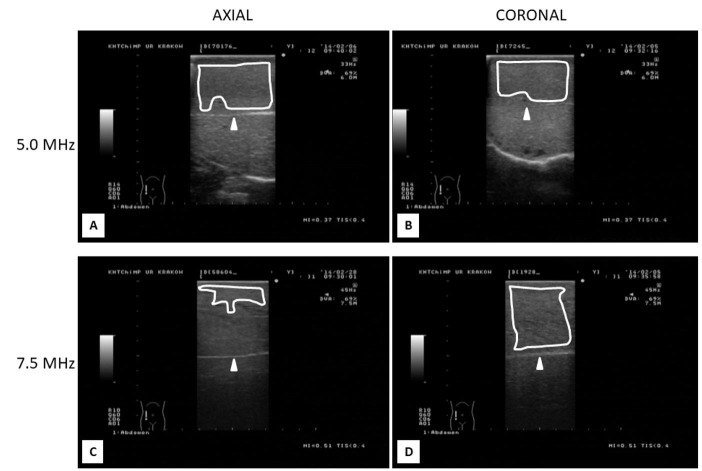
Photographic reproductions of mammary gland ultrasonograms from lactating ewes with the udder scanned in an axial (A and C) and coronal (B and D) plane using either 5.0- (A and B) or 7.5-MHz (C and D) linear-array transducers. White arrowheads indicate the location of the connective tissue septum separating the two halves of the udder. Delineated areas (white lines) represent the potential placement of computer-generated polygonal meters used for echotextural analyses of the mammary parenchyma (Image ProPlus
